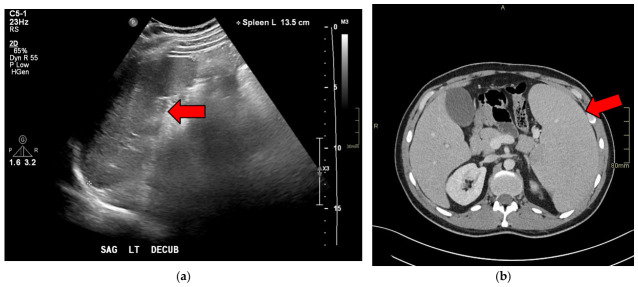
Lymphoma (a) On ultrasound, an enlarged spleen with hypo-echoic ill-defined lesions (b) On contrast-enhanced CT, the spleen is enlarged with homogenous attenuation.
